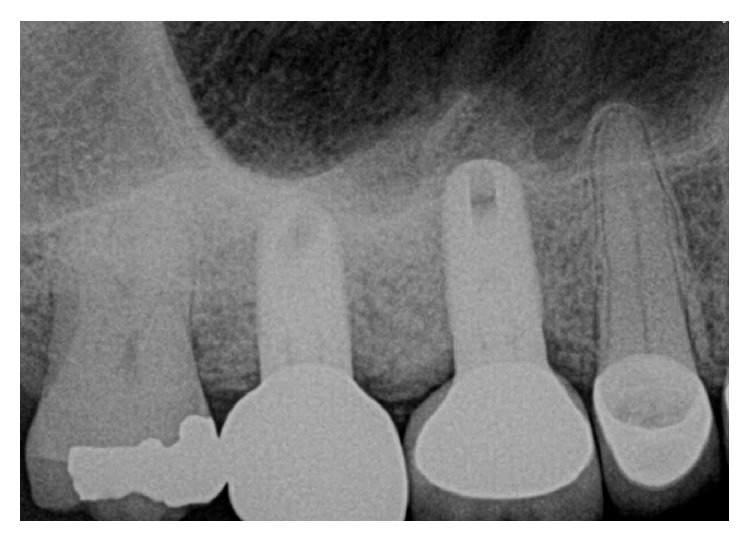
A periapical radiograph on the day of surgical debridement showing a 5 × 5 mm lack of bone density around the implant apex.
